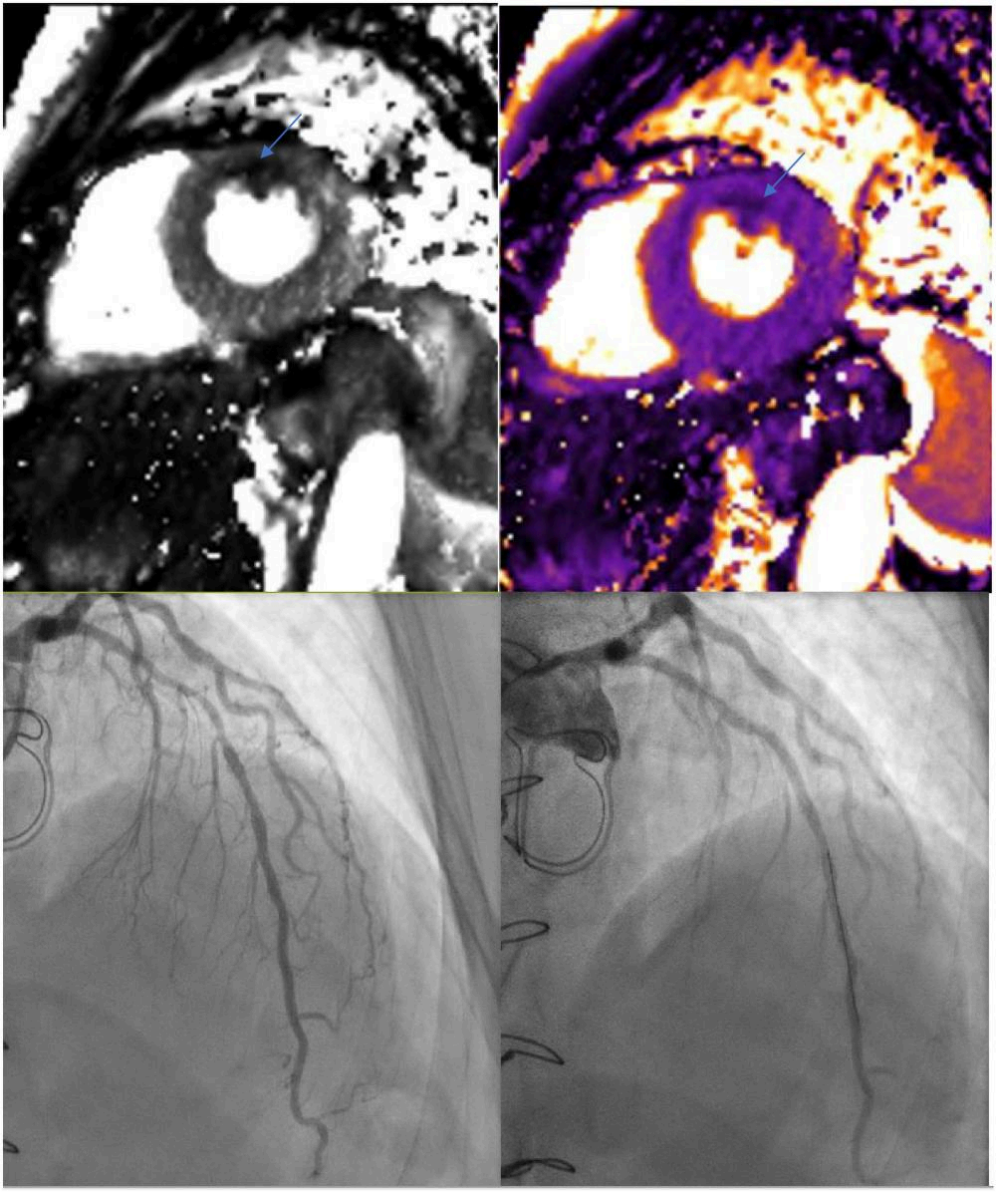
Multimodal imaging in a 75-year-old post-Bentall patient using stress MRI showing new anterior ischaemia (arrow) beyond prior necrosis due to evolving and ischaemic long LAD lesion, successfully treated by PCI.
